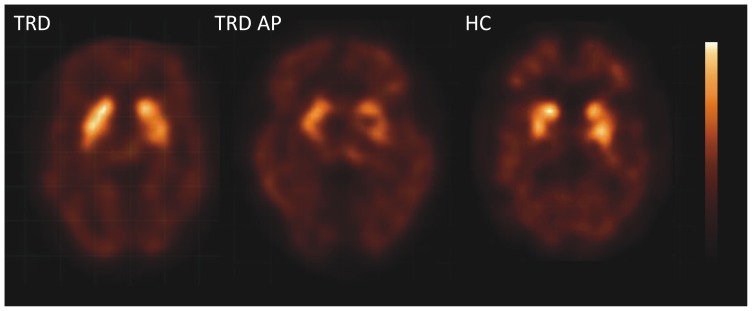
Transversal images of D2/3R availability. Transversal [123I]IBZM SPECT slices at the level of the striatum showing D2/3 receptor availability in a TRD patient, a TRD patient on antipsychotics (TRD AP), and a healthy control subject.
